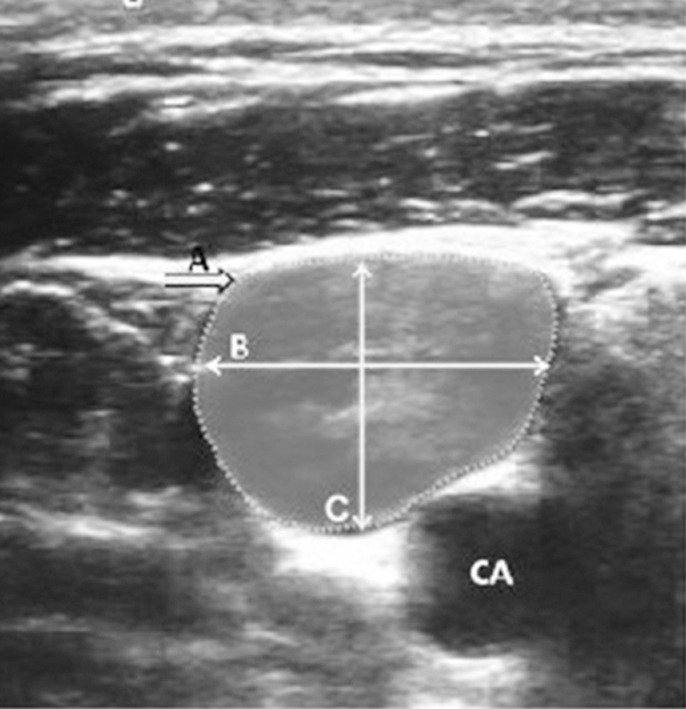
Ultrasound image of the right internal jugular vein and relationship with carotid artery (CA).
